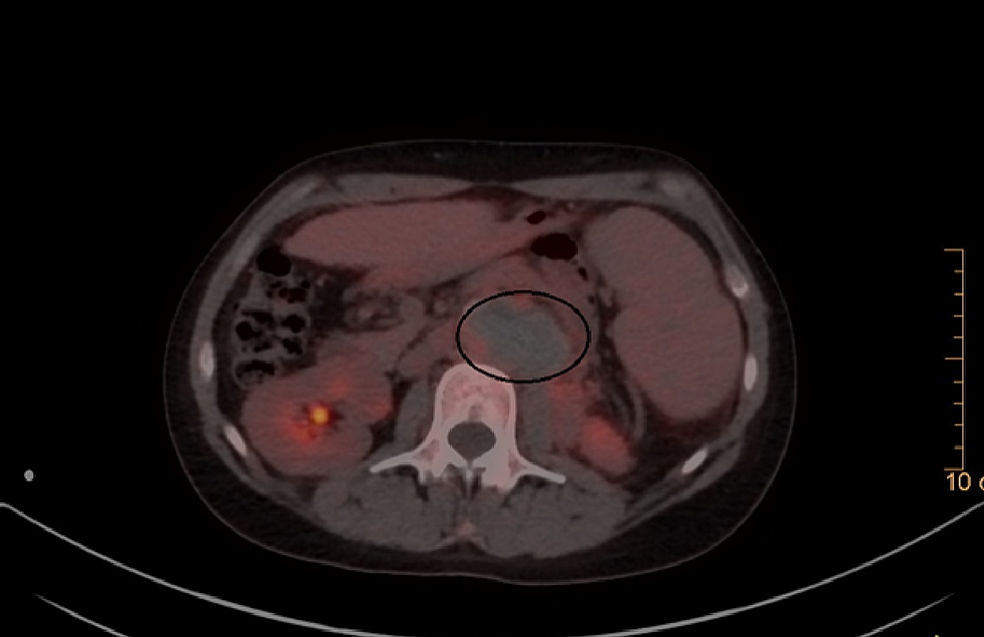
PET/CT with 18F-fluorodeoxyglucose (axial section) A left para-aortic oval image is described (highlighted by a black circle) with central hypometabolism and scarce peripheral metabolism, measuring approximately 55 * 33 mm in the axial plane, suggestive of a hematoma contained in the abdominal aorta. PET: positron emission tomography
